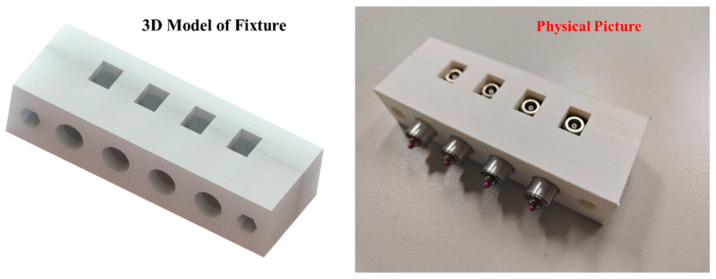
Ultrasonic array fixture.
